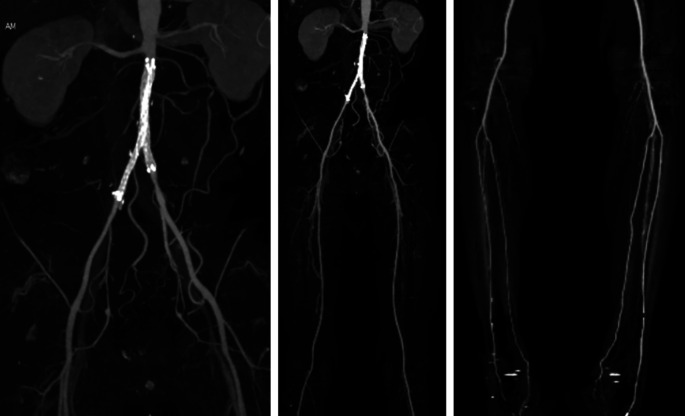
Post-procedural CT scan angiography.
